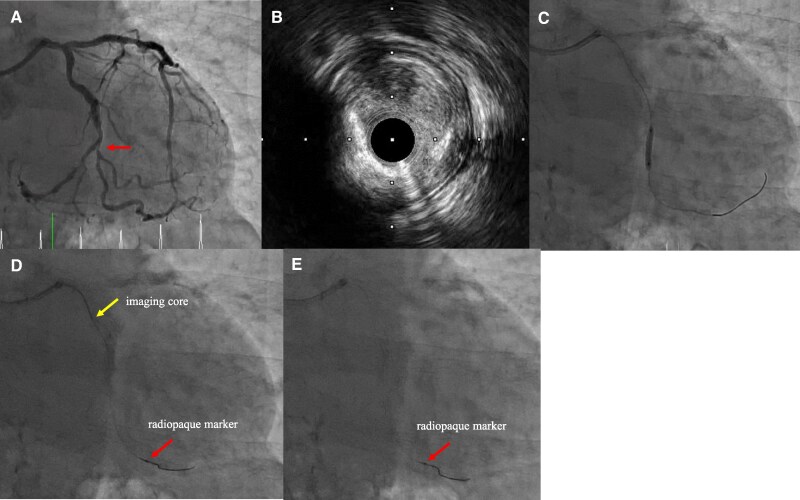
Coronary angiography and intravascular ultrasound findings. (*A*) The target lesion is shown with a arrow. (*B*) The intravascular ultrasound finding of the target lesion shows fibrous plaque with mild calcification. (*C*) Pre-dilatation of the target lesion using a balloon. (*D*) The intravascular ultrasound imaging core (arrow) is located abnormally distant from the radiopaque marker (arrow). (*E*) The radiopaque maker remains in the coronary artery after pulling out the main body of the intravascular ultrasound catheter.
